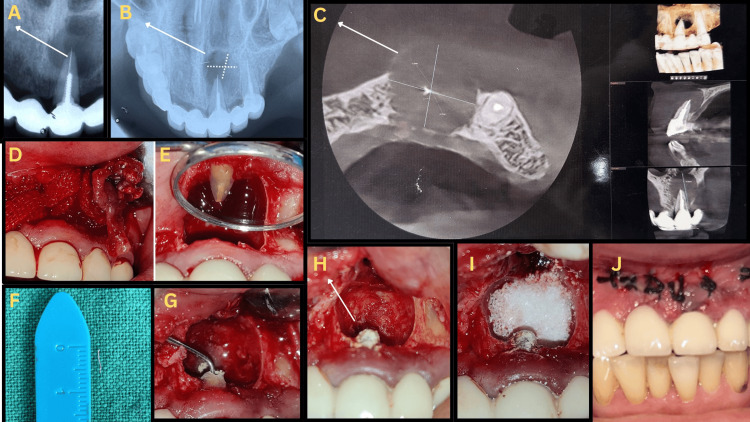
Treatment (A) Pre-operative intraoral periapical radiograph (IOPA) of tooth #21 showing a large periapical radiolucency with a separated instrument in the apical third; (B) occlusal radiograph (R/G) showing the extent of the lesion; (C) pre-operative cone-beam computed tomography (CBCT) (axial, coronal, and sagittal views) showing a 13.7 × 12.8 × 14.8 mm lesion with labial cortical discontinuity; (D) intra-operative view showing cyst enucleation; (E) visualized separated fragment; (F) retrieval of the fractured fragment; (G) apicoectomy and ultrasonic (US) retropreparation; (H) placement of Biodentine as the retrograde filling material; (I) placement of autologous platelet-rich fibrin (APRF) and allograft; and (J) suturing completed.
